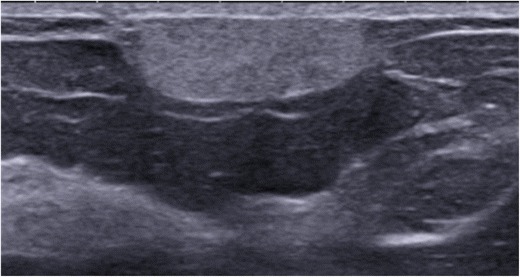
Angiolipoma. Transverse ultrasound scan of the right breast showing a homogeneous, hyperechoic, oval-shaped subcutaneous mass with circumscribed margins
